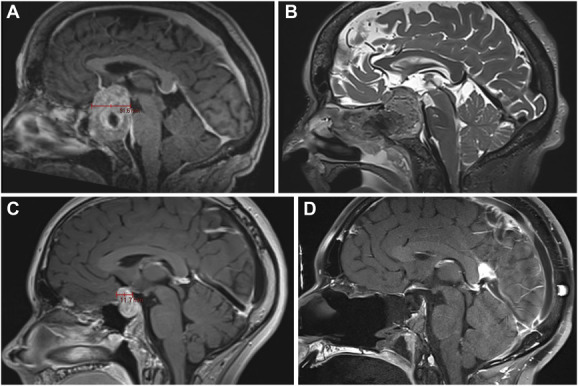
A, Contrast-enhanced sagittal T1-weighted preoperative MRI demonstrating a large PitNET with a suprasellar AP diameter of 31.5 mm. B, T2-weighted intraoperative MRI demonstrating central debulking of the tumor, with a significant suprasellar residual component requiring operative reintervention. C, Contrast-enhanced sagittal T1-weighted preoperative MRI demonstrating a PitNET with suprasellar extension and a suprasellar AP diameter of 11.7 mm. D, T1-weighted intraoperative MRI demonstrating gross total resection. AP, anterior-posterior; PitNET, pituitary neuroendocrine tumor.
